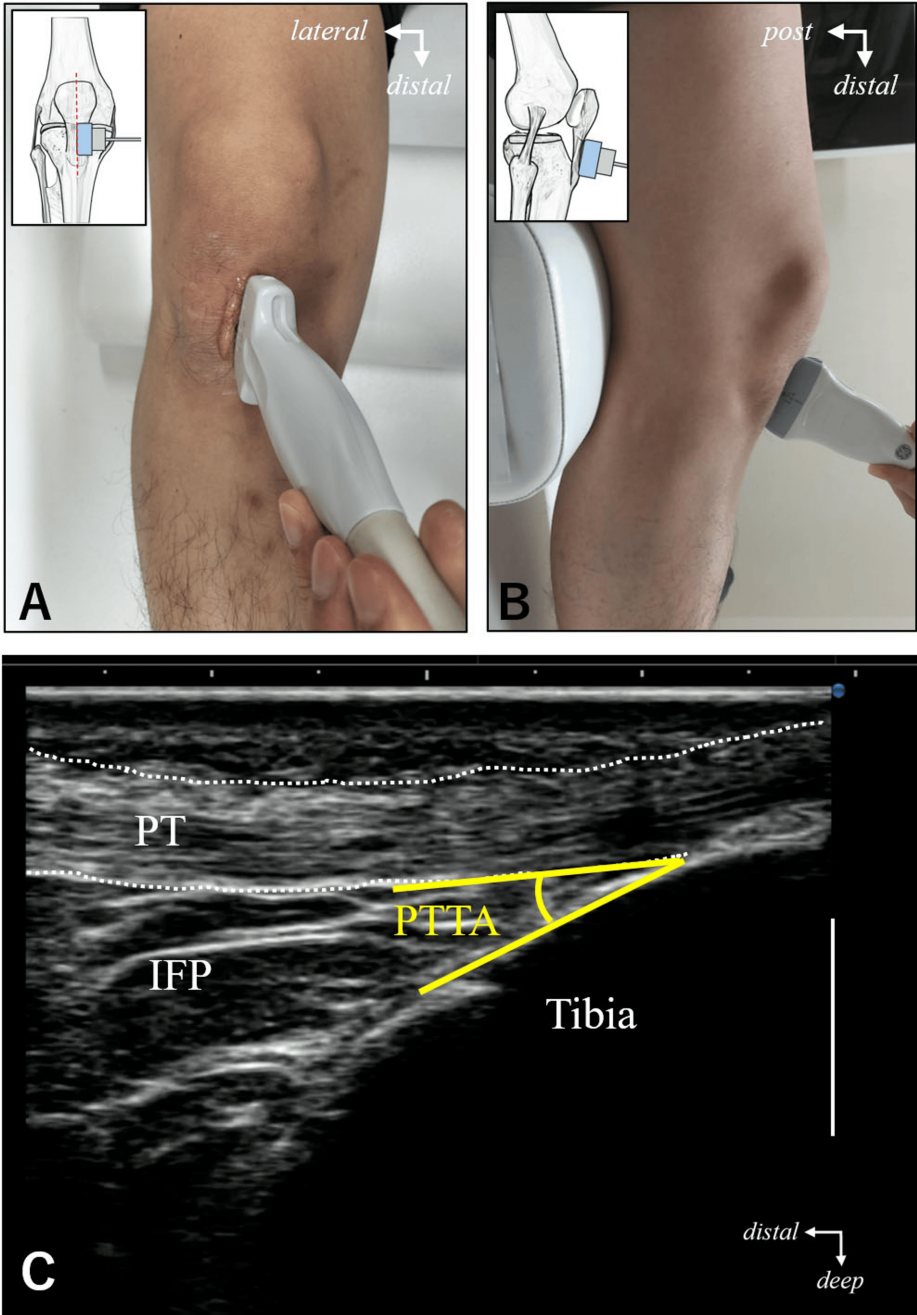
A method for ultrasound imaging of the infrapatellar fat pad at the anterior aspect of the proximal tibia. A and B are the position of the probe when measuring. A: anterior view; B: lateral view; C: ultrasound image. The angle composed of the deep border of the patellar tendon and the anterior surface of the proximal tibia was defined as the patellar tendon-tibia angle and measured. PT, patellar tendon; IFP, infrapatellar fat pad; PTTA, patellar tendon-tibia angle. Scale bar 10 mm.
